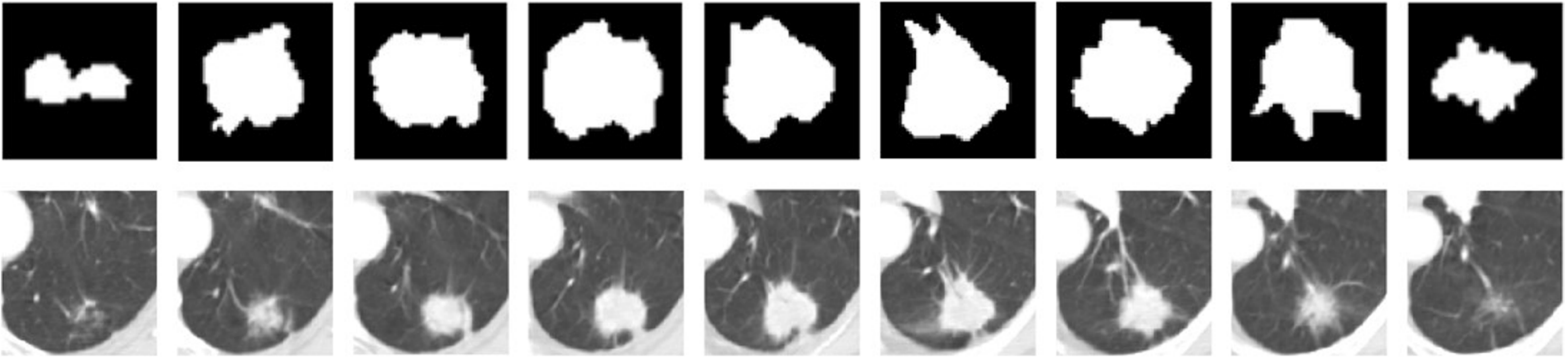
Images (top row) and the corresponding binary shapes (bottom row) of a nodule selected from a series of CT slices
